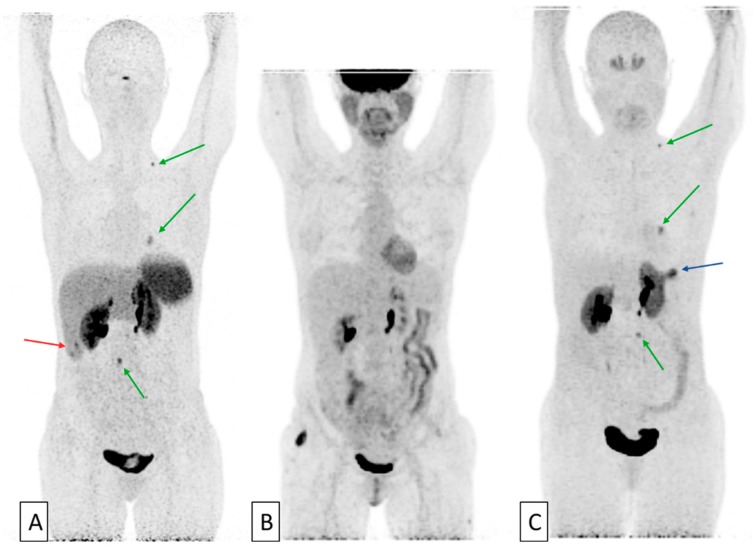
An example of a 46-year-old patient with pluri-metastatic intestinal neuroendocrine tumor (grade 2, ki67 at 4%). 68Ga DOTA-TOC (A), FDG-PET (18F-fluoro-deoxyglucose- positron-emission-tomography) (B) and FDOPA (18F-fluorodihydroxyphenylalanine) (C) imaging were realized. MIP (maximum intensity projections) images from the respective PET data sets are shown. The subject has positive on both FDOPA and somatostatine-receptor imaging, dominant disease which exhibits no FDG uptake (green arrows). One hepatic lesion was FDOPA-negative and 68Ga-DOTA-TOC-positive (red arrow) and one gastric lesion was FDOPA-positive and 68Ga-DOTA-TOC negative (blue arrow). Images courtesy of Pr C. Bodet-Milin.
